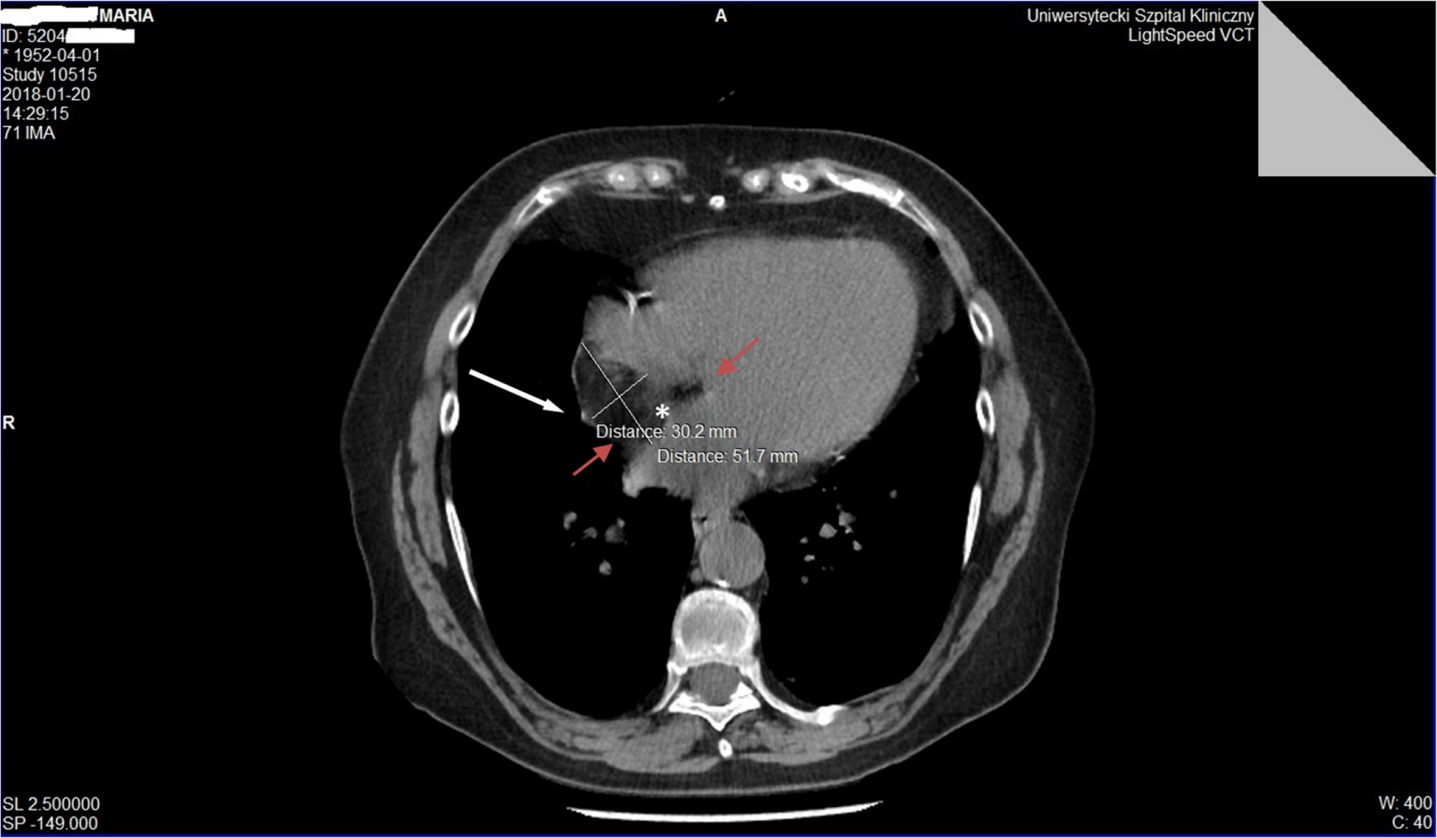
CT scan. Visible lipomatous tissue surrounding the fossa ovalis (*) with the characteristic hourglass shape (between the red arrows). The marked mass, measuring 30.2 mm × 51.7 mm, extends to the back wall of the right atrium (RA) and crista terminalis (white arrow)
